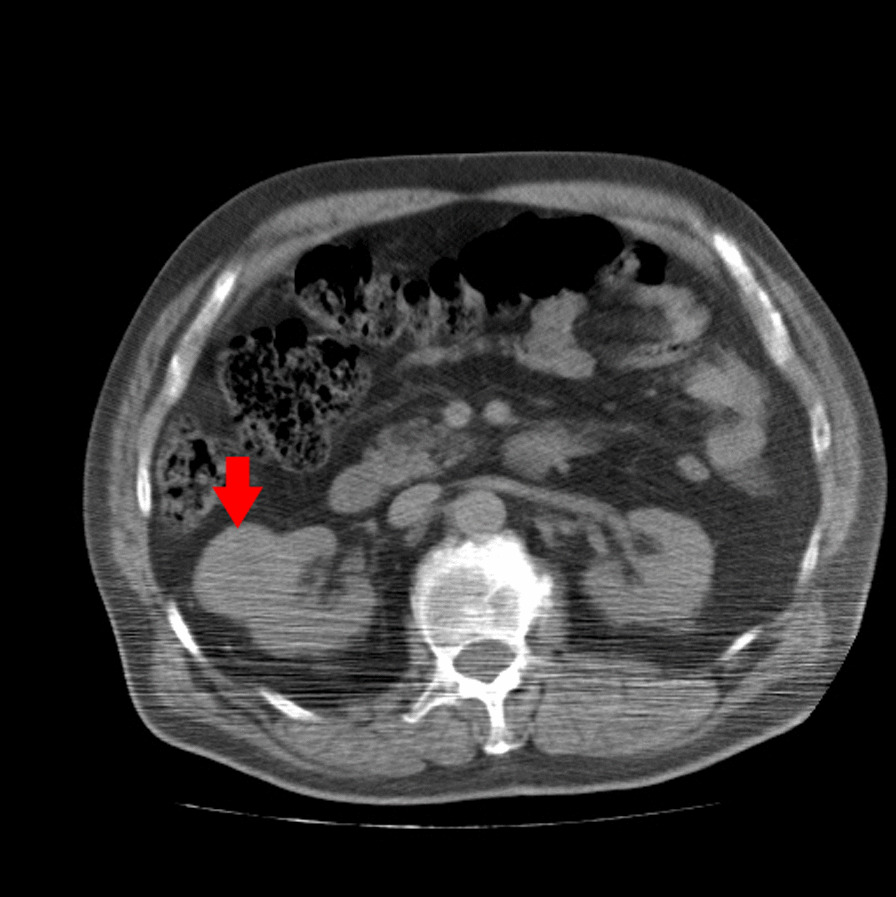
Axial computed tomography of the abdomen and pelvis revealing well-defined mass measuring approximately (45 × 40) mm arising from the lateral aspect of the right kidney
